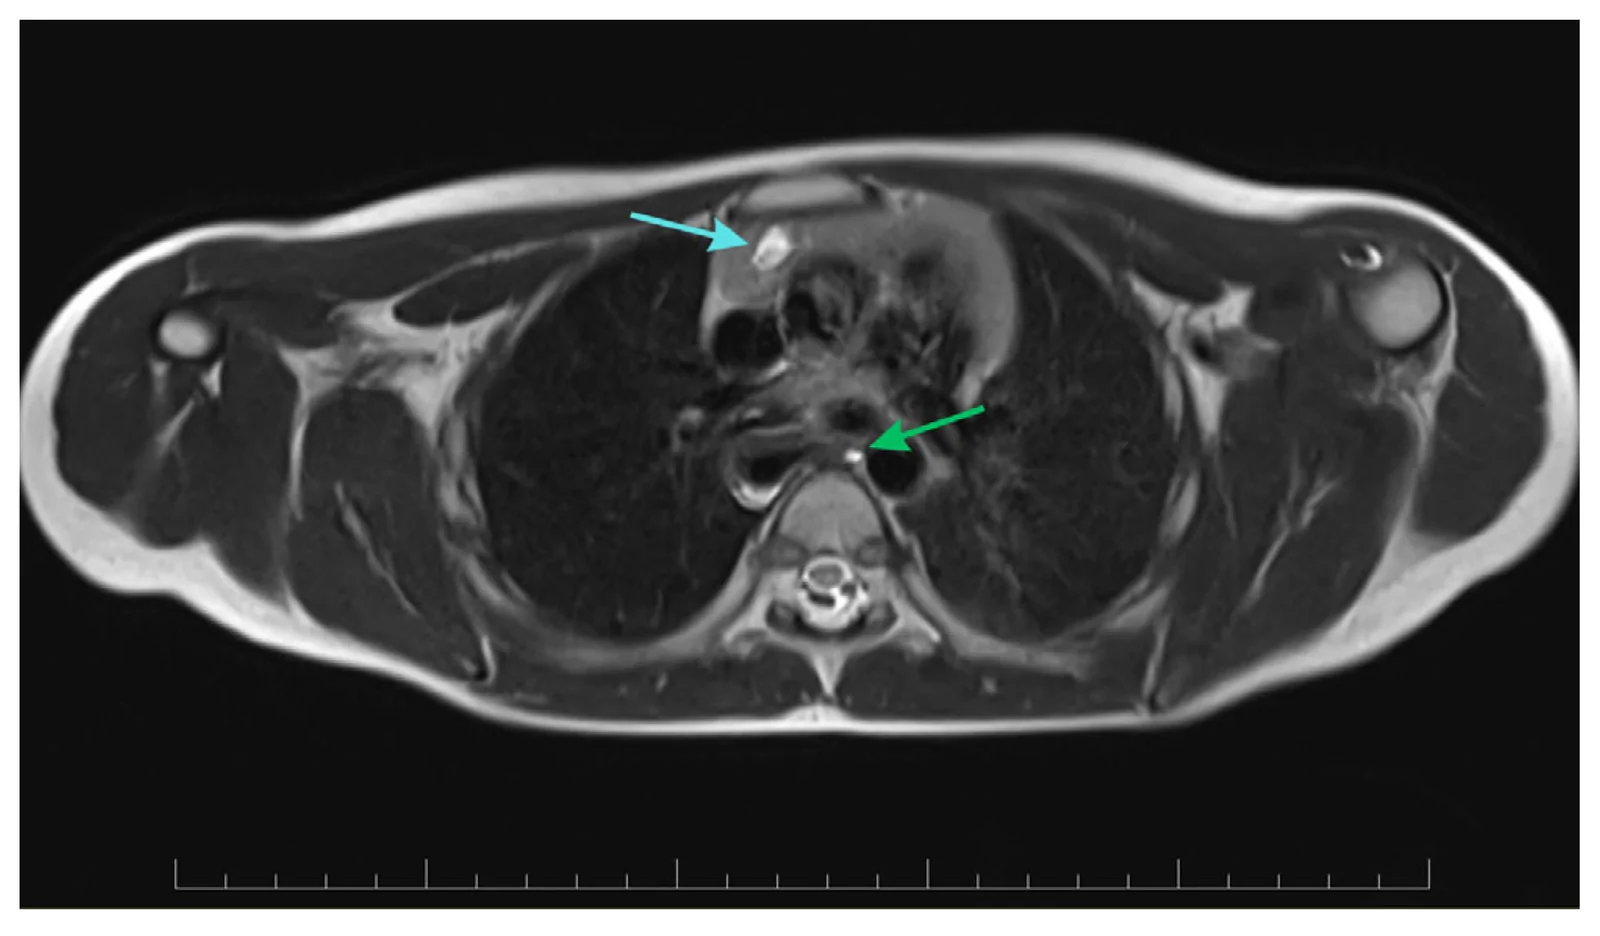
Thoracic MRI of a 6-year-old male, demonstrating a septated cystic formation in the right thymic region (blue arrow) suggestive of mediastinal lymphangioma, diffuse esophageal dilatation along its entire length and a small cystic image (green arrow) adjacent to the esophageal wall.
